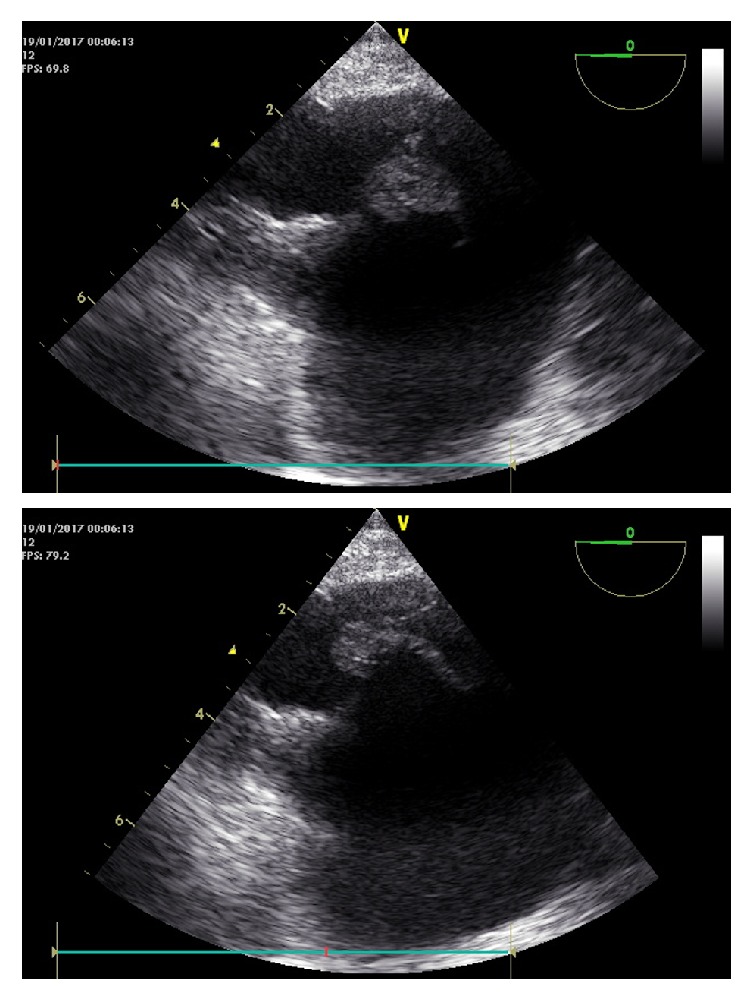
Transesophageal echocardiogram, multilobular heterogeneous vegetation attached to Eustachian valve.
